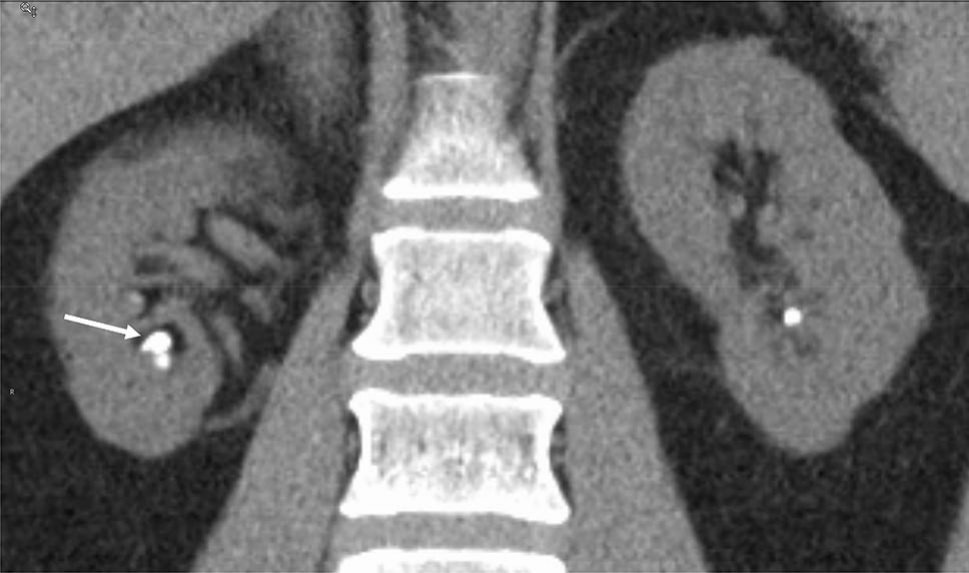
CT scan demonstrating a loose intracalyceal stone in the right kidney (arrow) and papillary calcifications in both kidneys
